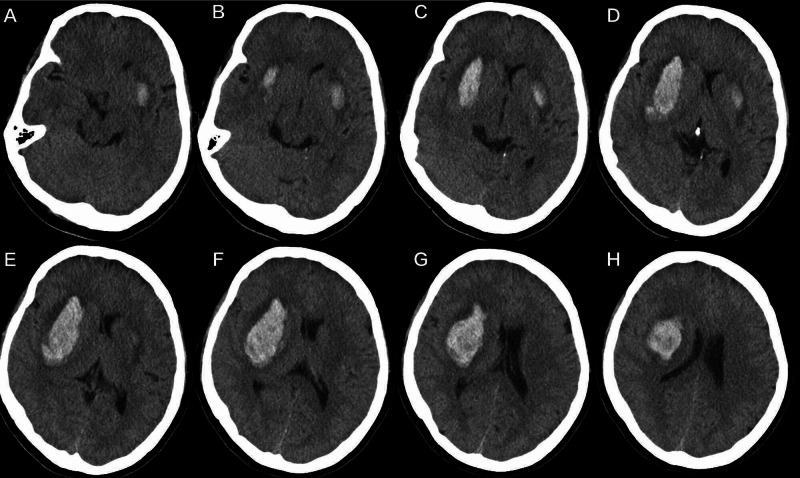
Preoperative axial CT of the patient. A-H: Preoperative axial CT of the patient. It shows bilateral intraparenchymal hemorrhages, mainly in the putaminal area.
